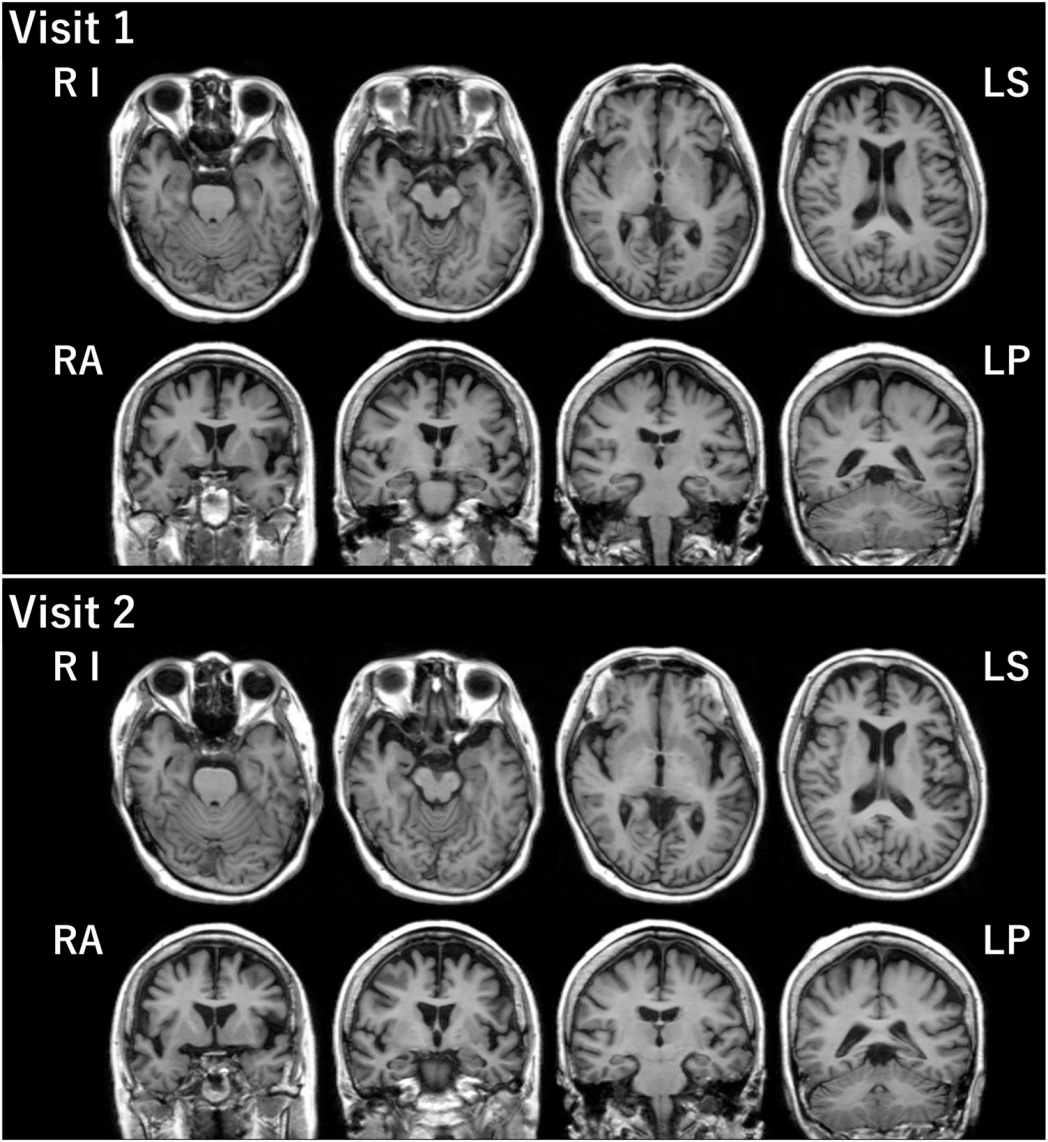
Brain MRI. Brain MRI at visits 1 and 2 revealed relative preservation of the medial temporal lobe and left-sided predominant mild atrophy in the bilateral perisylvian area. LP, left posterior; LS, left superior; RA, right anterior; RI, right inferior.
